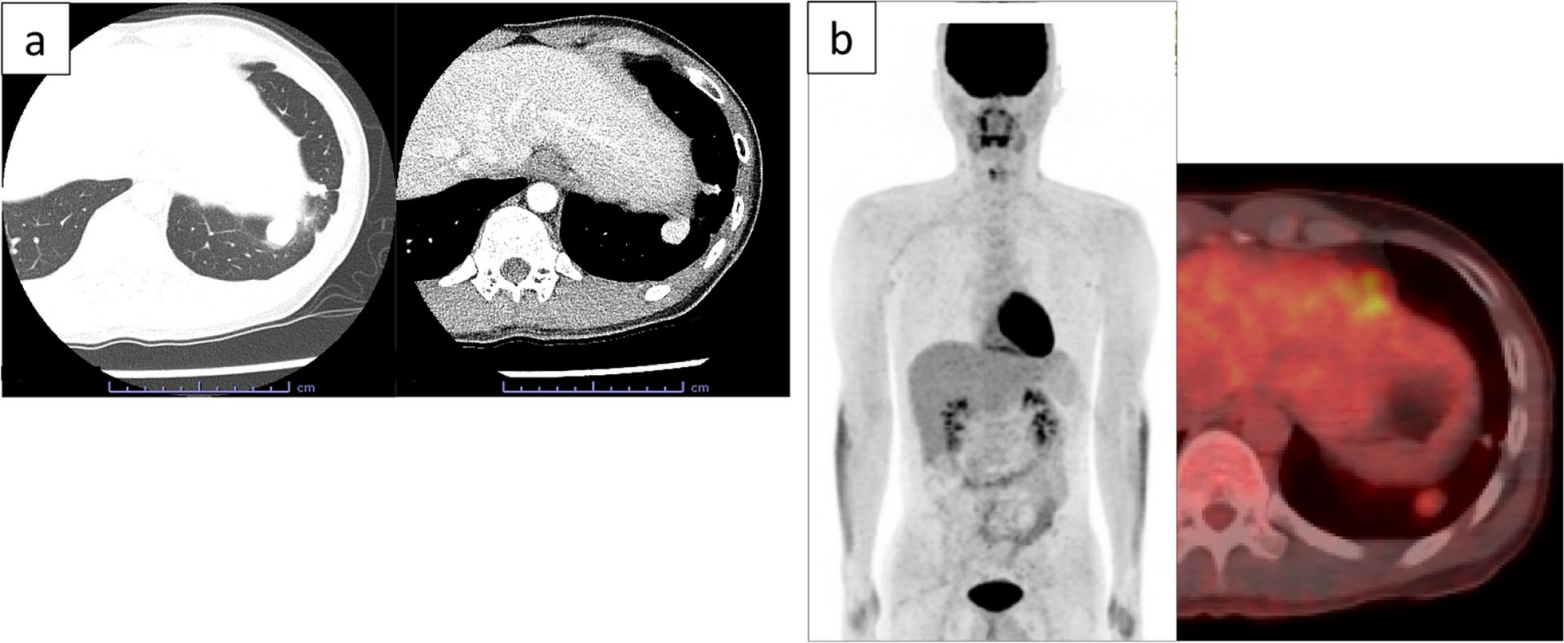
Two new lesions above the diaphragm were found on chest CT at 1 year and 6 months after the first surgery (a). FDG-PET showed that the SUVmax intake of the tumors was 2.32, and metastasis to other organs was not found (b)
